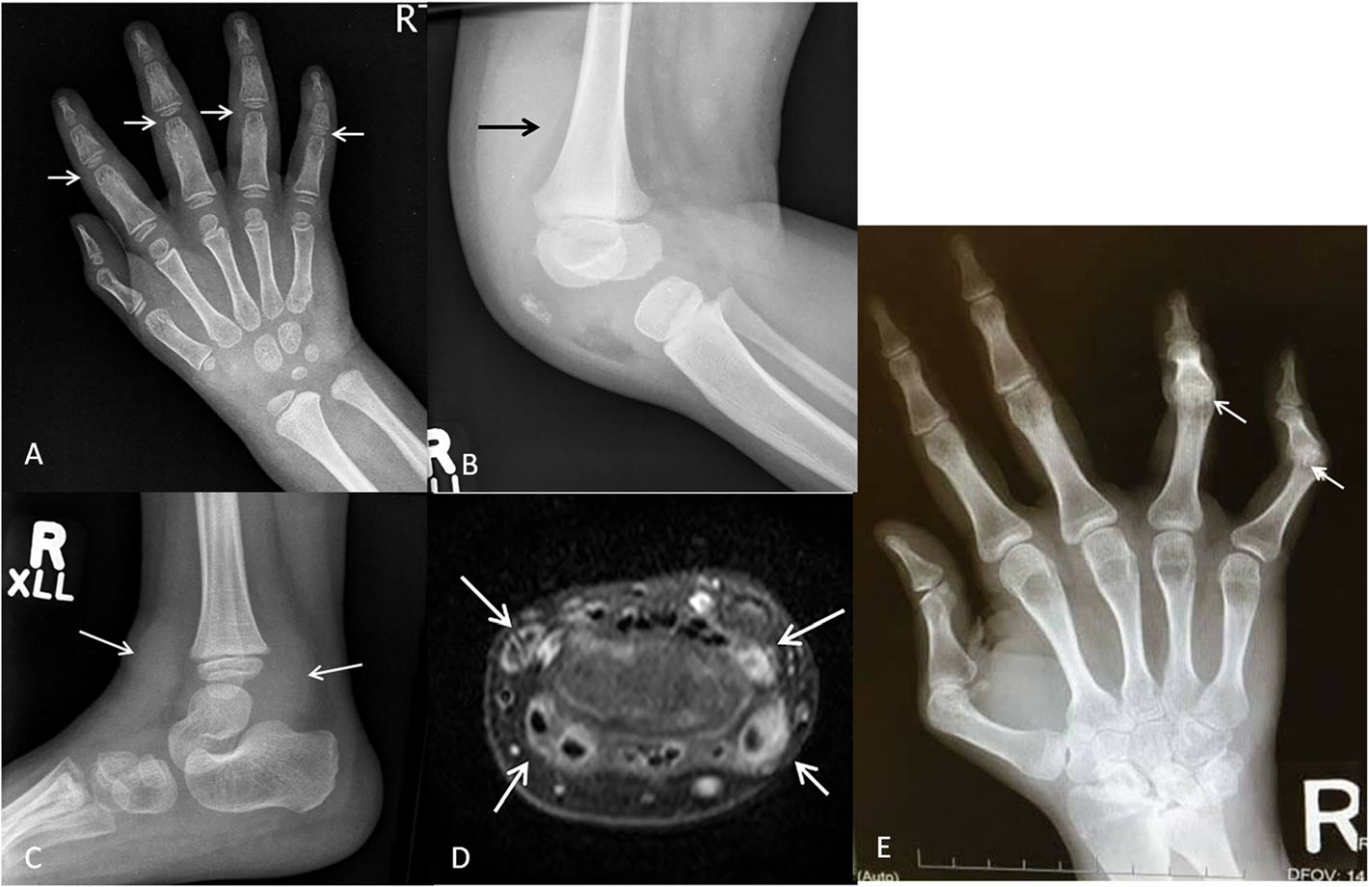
A 2-year-old girl with Blau syndrome presenting with swelling of the hands, knees, and ankles. a Frontal radiograph of the right hand demonstrates diffuse periarticular osteopenia, soft tissue swelling around the proximal interphalangeal joints (arrows). No joint space narrowing, erosions or osseous destruction. Similar changes were present in the left hand (not shown). b Lateral radiograph of the right knee demonstrates large suprapatellar joint effusion (arrow) and soft tissue swelling. Similar changes were seen in the left knee (not shown). c Lateral radiograph of the right ankle demonstrates soft tissue swelling along the tendons representing tenosynovitis (arrows). d Axial T1-weighted image with fat suppression after intravenous contrast administration of the right wrist demonstrate thickening and enhancement of the tendon sheaths of all the extensor tendons (arrows) representing tenosynovitis. e follow up frontal radiograph of the right hand of the same patient at the age of 25 years demonstrates typical flexion contracture of the PIP joints of the fourth and fifth digits (arrows). Note that there are no erosions
